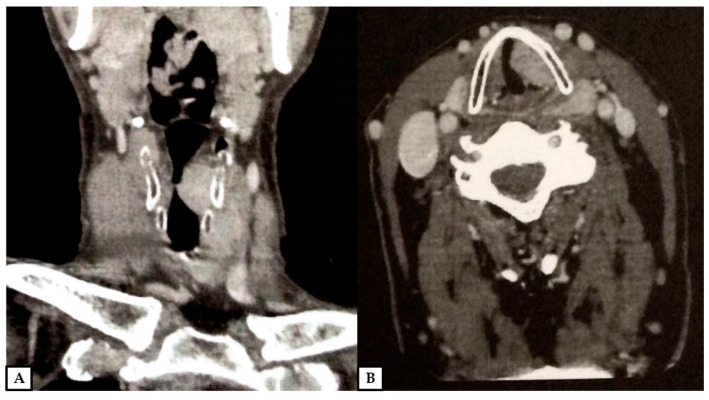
A 66-year-old man presented with a previous diagnosis of granular cell tumor of the left vocal cord on an incisional biopsy performed at another hospital. Contrast-enhanced computed tomography revealed a soft tissue mass of 2.8 × 2.5 × 1.1 cm of the left vocal cord, with transglottic extension, spreading to the supraglottis, subglottis, paraglottic space and the anterior commissure, causing partial obstruction of the laryngeal lumen; no infiltration of the cartilage was evident (computed tomography scans; (A): coronal plane, (B): axial plane; previously unpublished, original photos).
